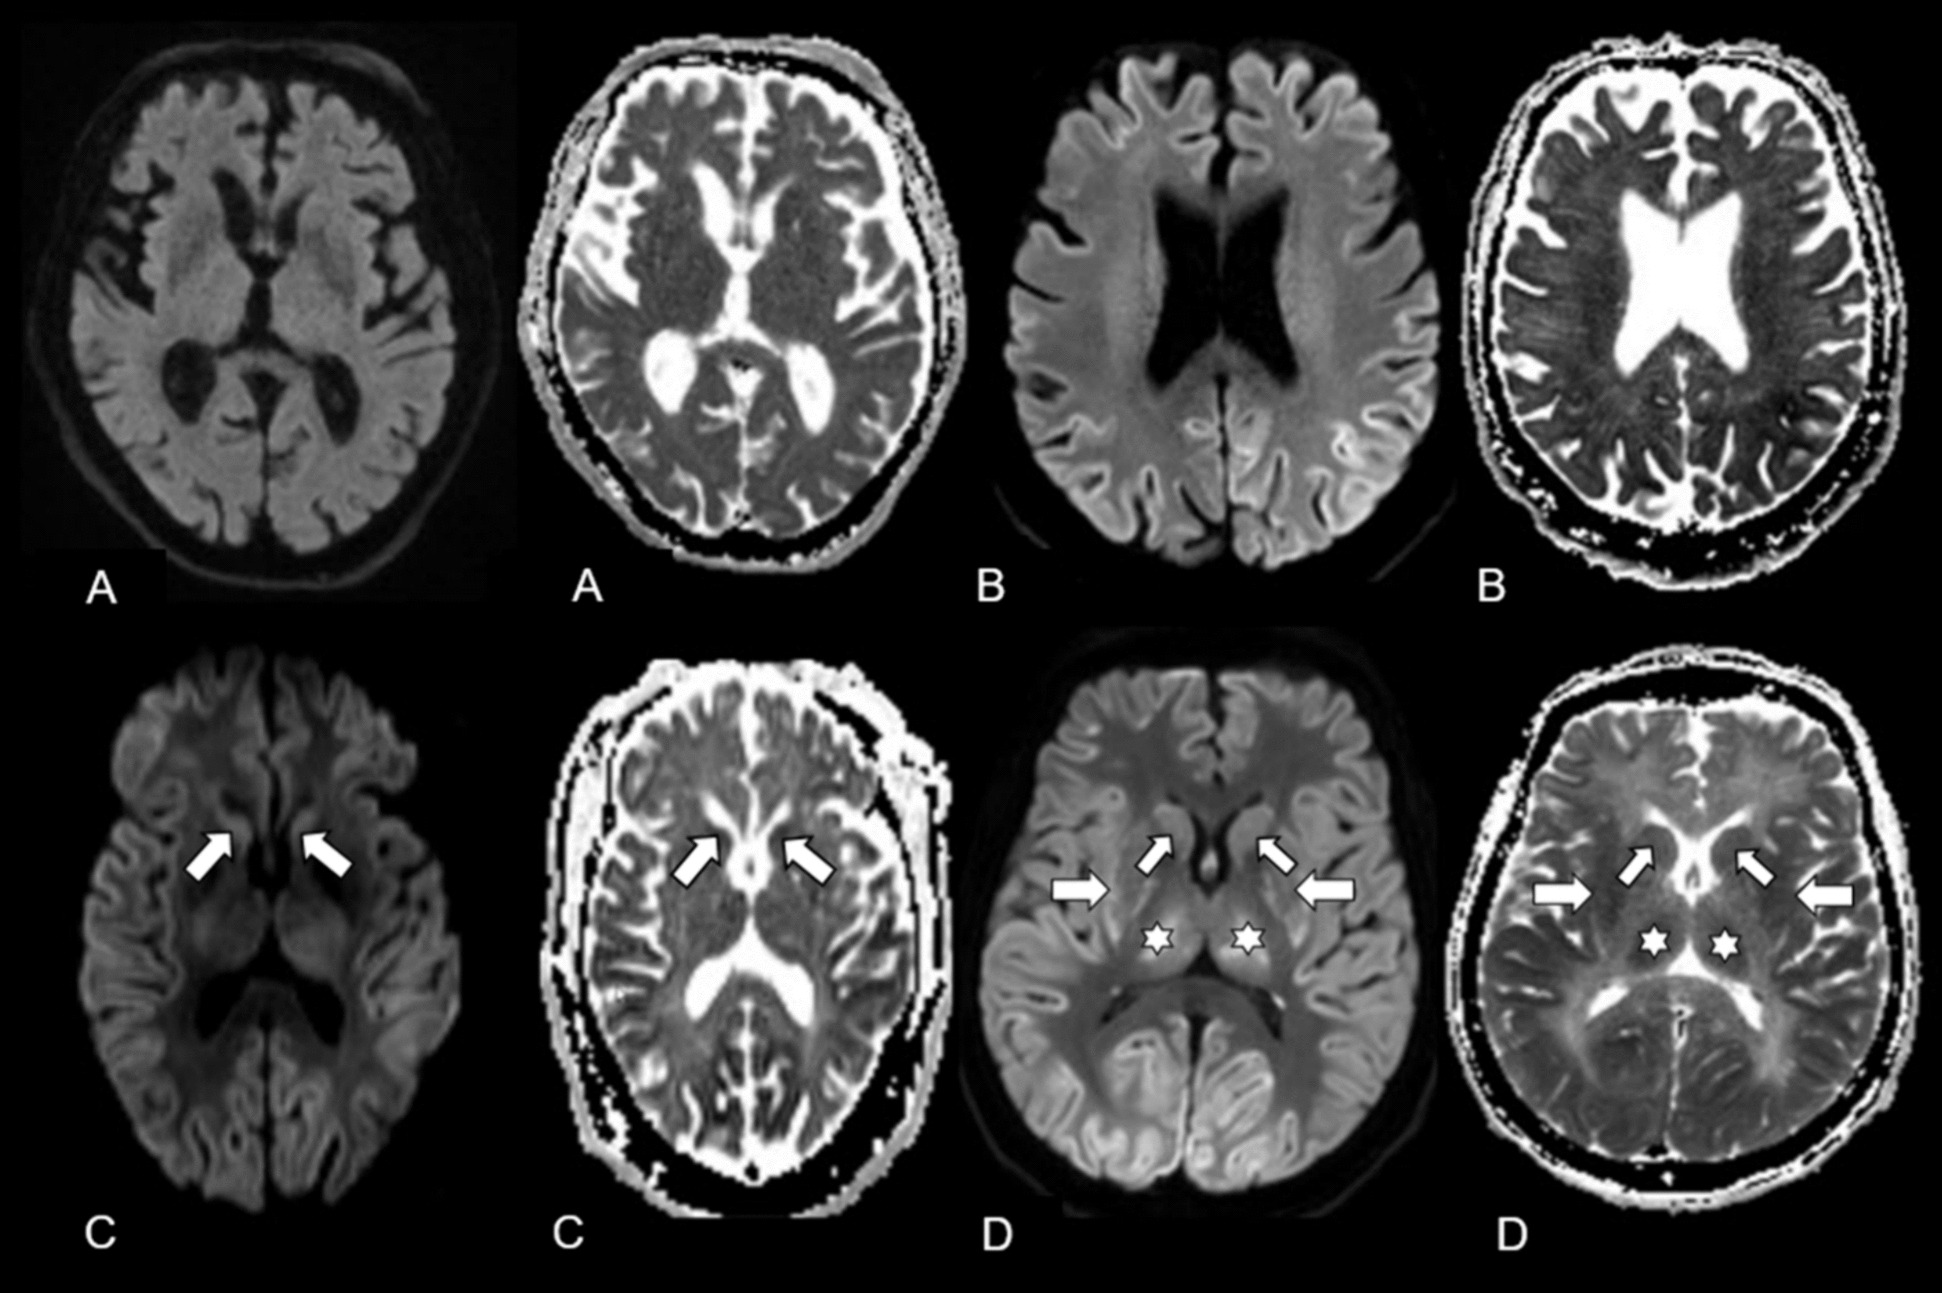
Representative examples for the four MRI lesion pattern (MLPs)
